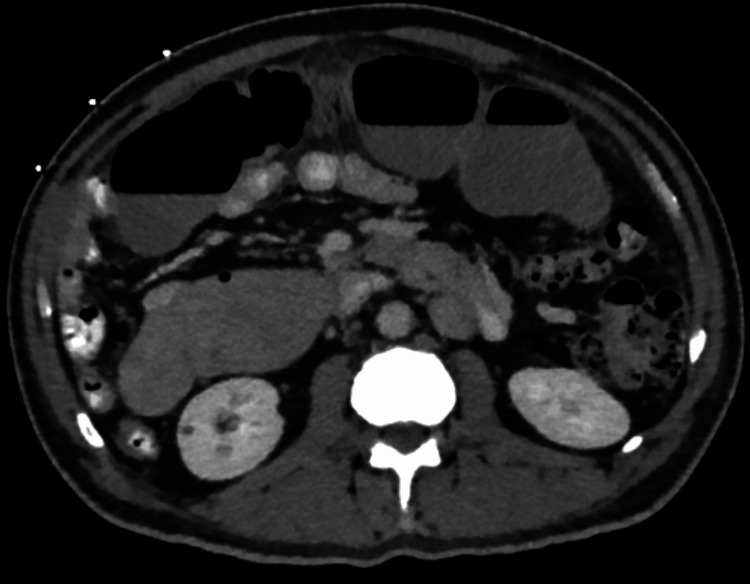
Axial contrast-enhanced CT image demonstrating markedly dilated proximal small-bowel loops with an abrupt caliber change, findings consistent with recurrent high-grade small bowel obstruction.
